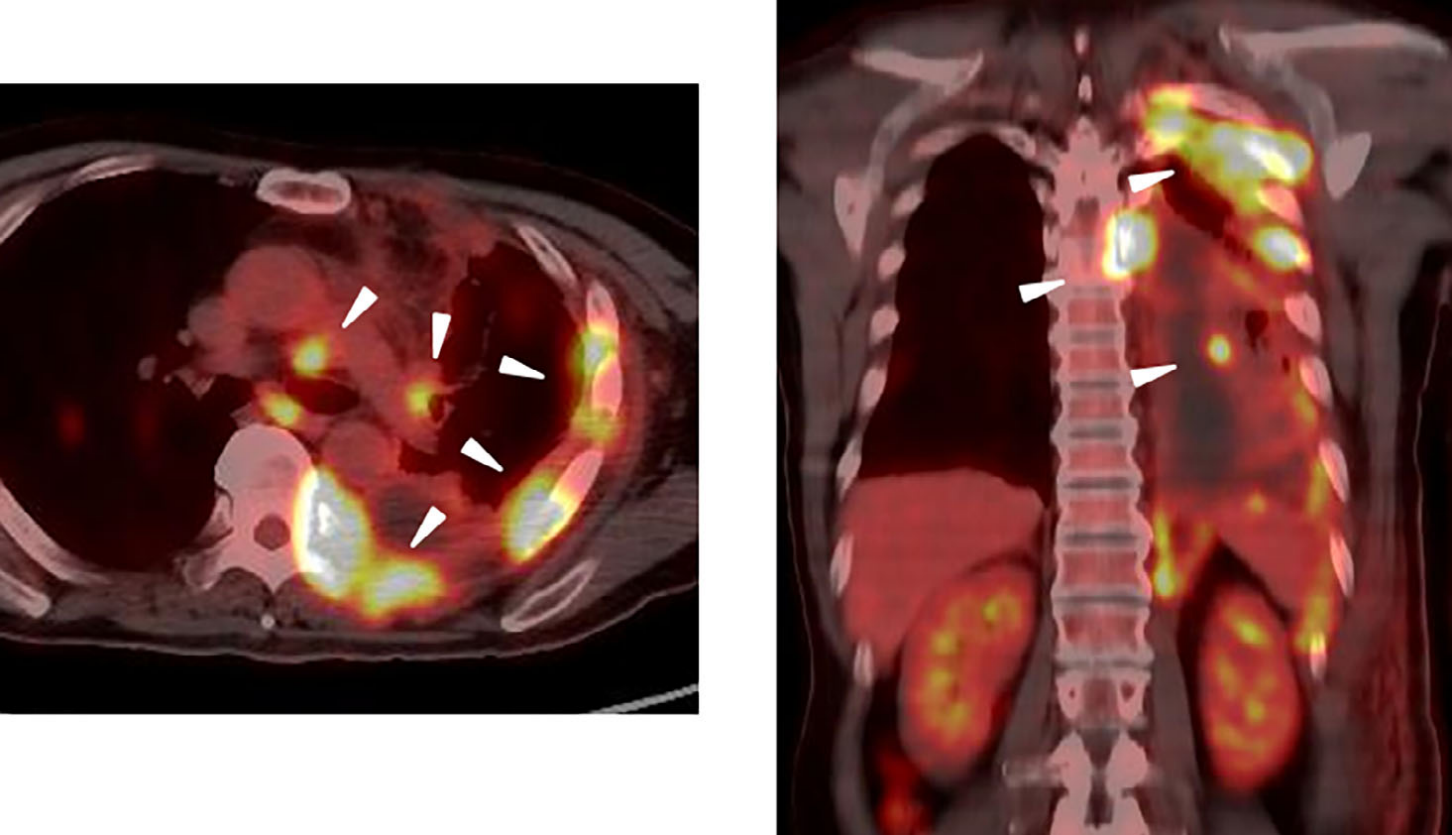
PET‐CT examination before Nivolumab/Ipilimumab combination therapy. Fluorodeoxyglucose accumulation was observed in the left pleura, lung field, and hilar lymph nodes. No obvious metastatic foci were noted in the liver.
